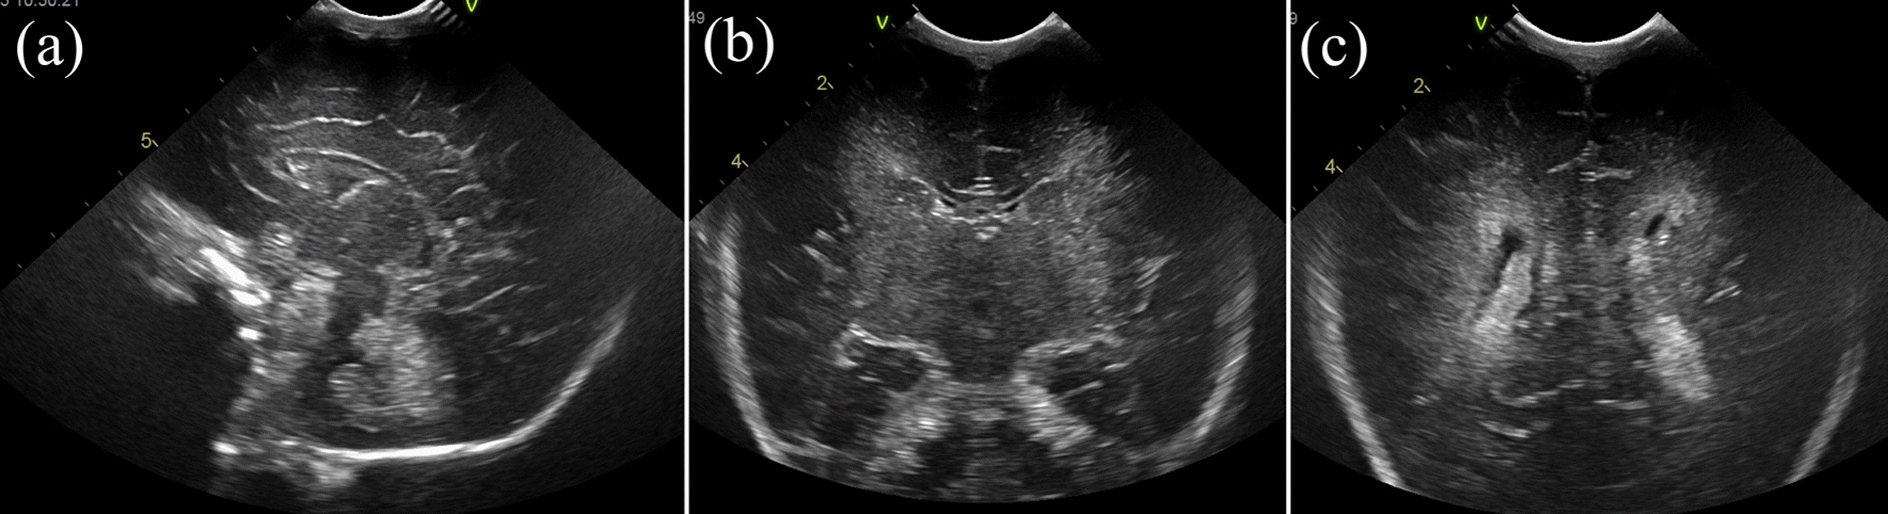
Cranial ultrasound at 3 hours after birth: a sagittal view, b coronal view (anterior horn of lateral ventricles), and c coronal view (posterior horn of lateral ventricles). No hyperechoic lesions indicative of hemorrhage observed
